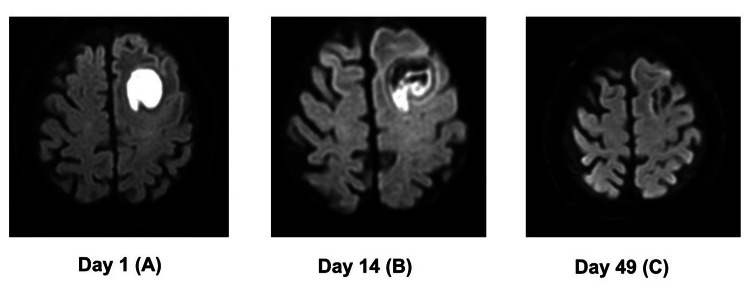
Changes in head MRI. (A) Preoperative head MRI showing an abscess in the left frontal lobe. (B) Postoperative image. The brain abscess decreased in size following drainage and antimicrobial therapy. However, a residual abscess remained. (C) Final MRI scan. The clearance of the abscess was confirmed following 45 days of MNZ therapy and further drainage. MRI: magnetic resonance imaging; MNZ: metronidazole
